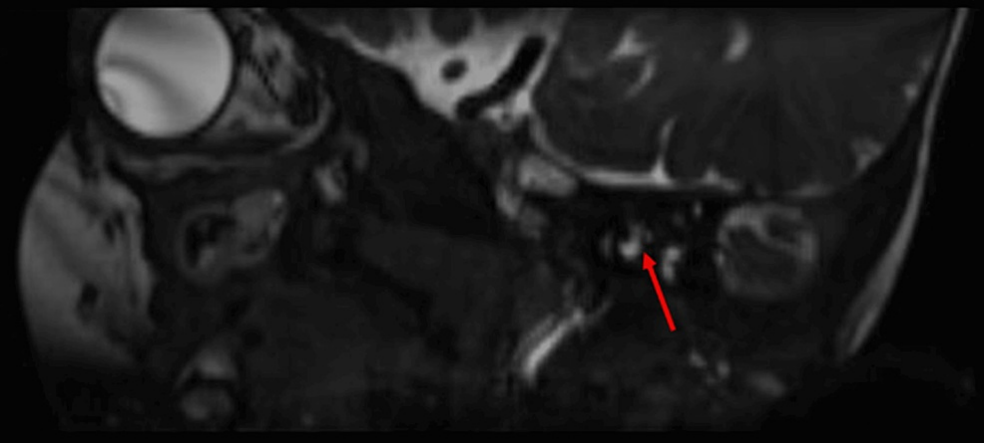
Sagittal sections of the CISS sequence of the MRI of the internal auditory canal on the left side reveal a normal eighth nerve with the absence of the seventh nerve. CISS: constructive interference in steady state
